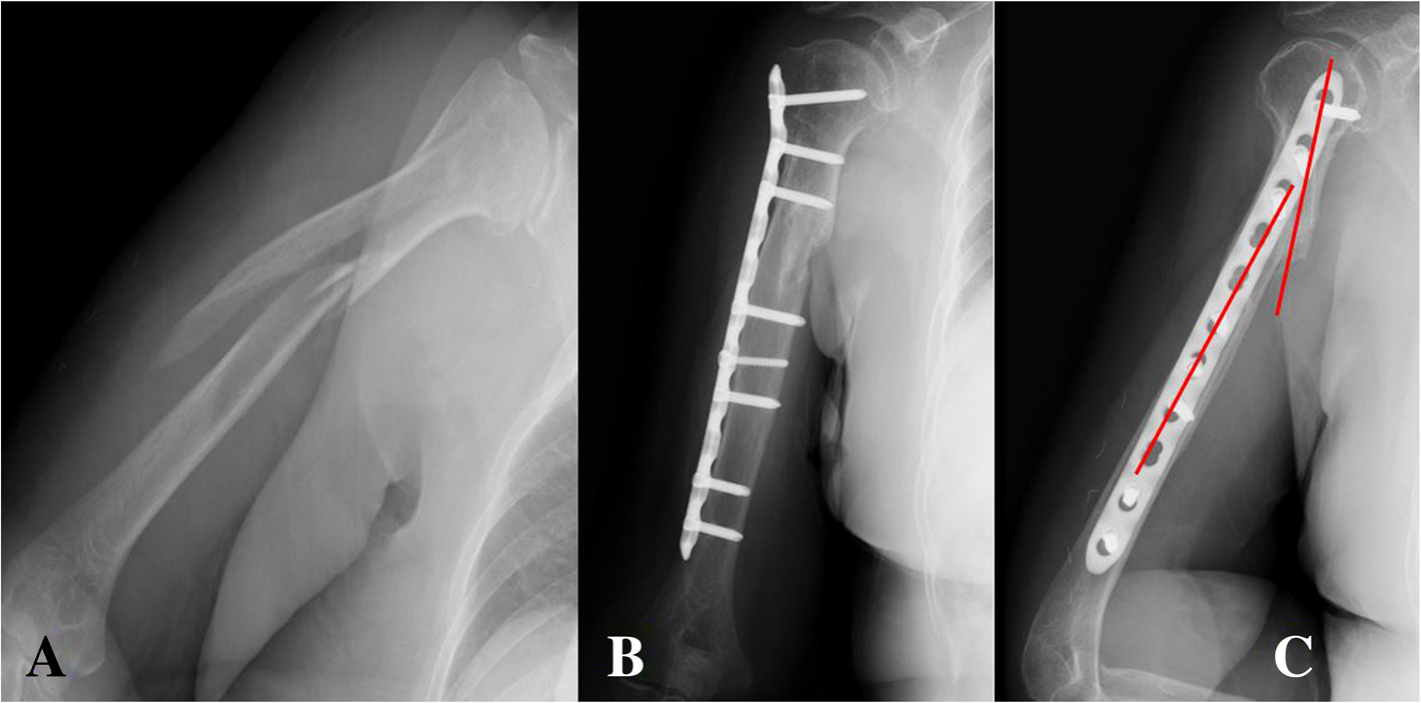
In MIPO technique using the narrow locking compression plate, intraoperative bending of this locking plate could adequately fitted to long spiral (Complex spiral type, AO classification: C1) humerus shaft fracture with proximal fracture extension to the greater tuberosity (a). However, at the postoperative lateral x-ray, the fracture angulation could be found after non-anatomical narrow locking compression plate fixation (b, c)
